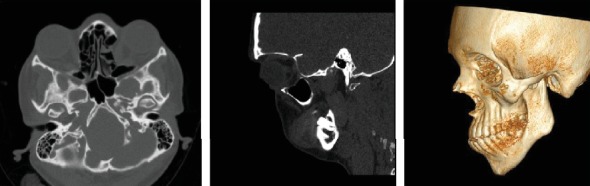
Pretreatment axial, sagittal, and three-dimensional computed tomographic views.
